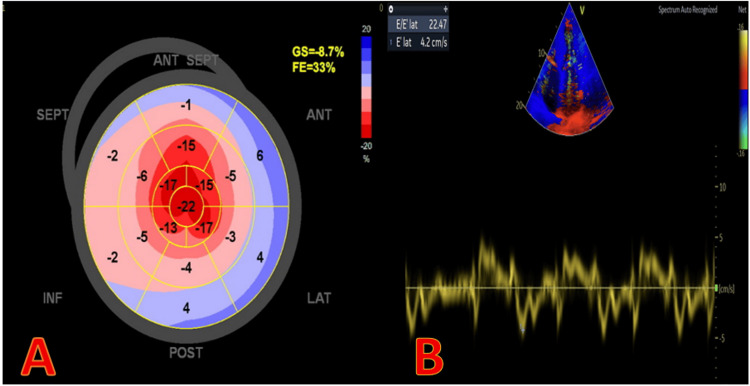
Functional echocardiographic findings suggestive of CA Functional echocardiographic assessment demonstrating characteristic findings of CA. (A) Left ventricular global longitudinal strain analysis showing severely impaired systolic function (GLS: −8.7%) with a typical apical sparing pattern on the bull's-eye map. (B) Tissue Doppler imaging demonstrating elevated left ventricular filling pressures with an E/e′ ratio of 22.47, consistent with restrictive left ventricular diastolic dysfunction. CA: cardiac amyloidosis
